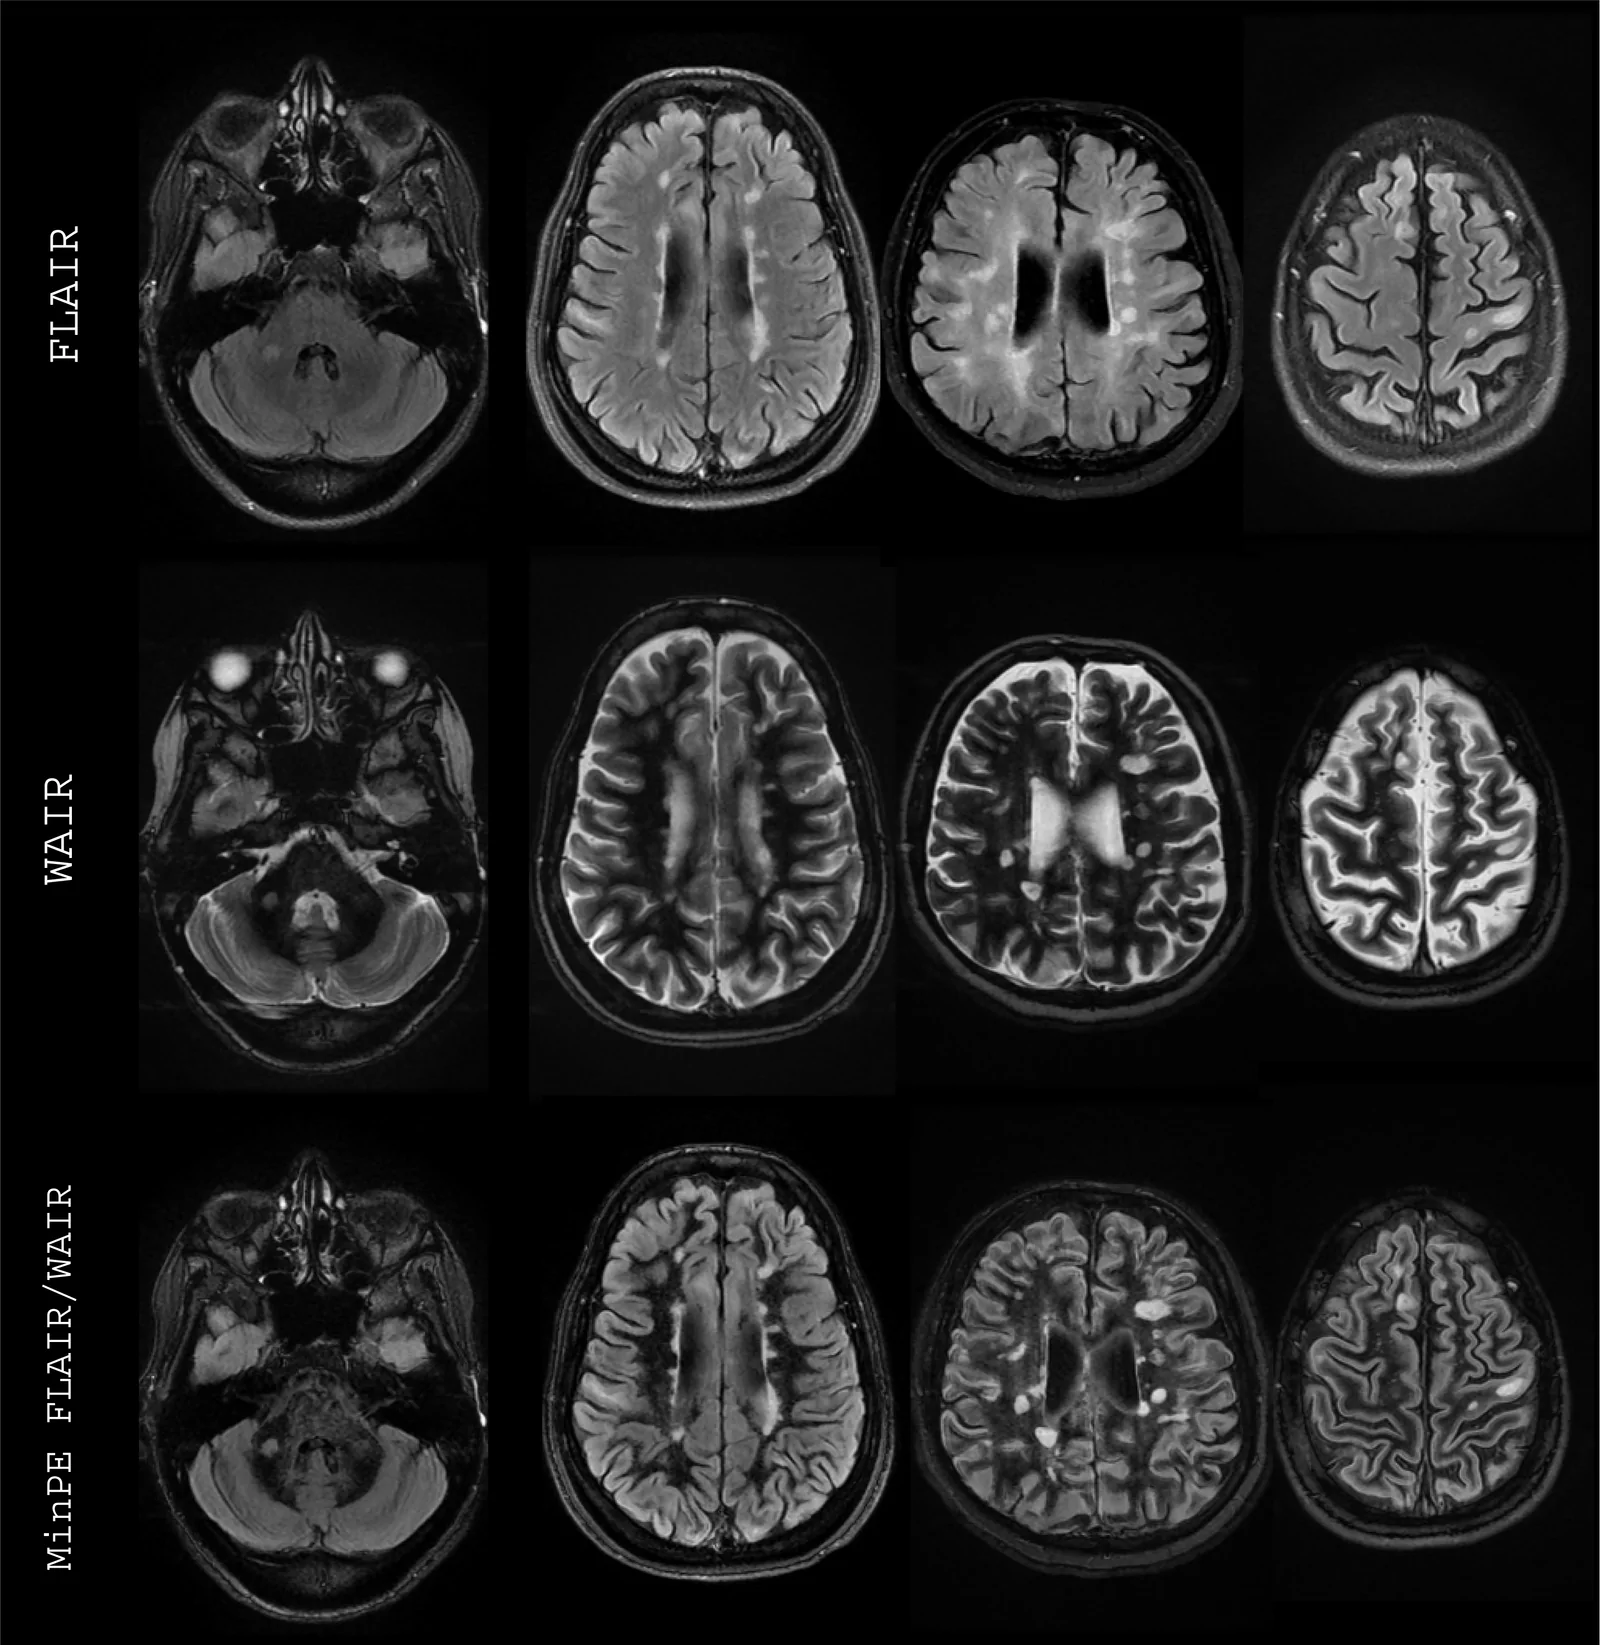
Example of combining FLAIR and WAIR images to generate a MinPE FLAIR/WAIR image. The MinPE FLAIR/WAIR images were generated by fusing the minimum pixel value extraction, which is an operation that consists of finding the minimum image intensity values pixel by pixel in both the FLAIR and WAIR sequences.
